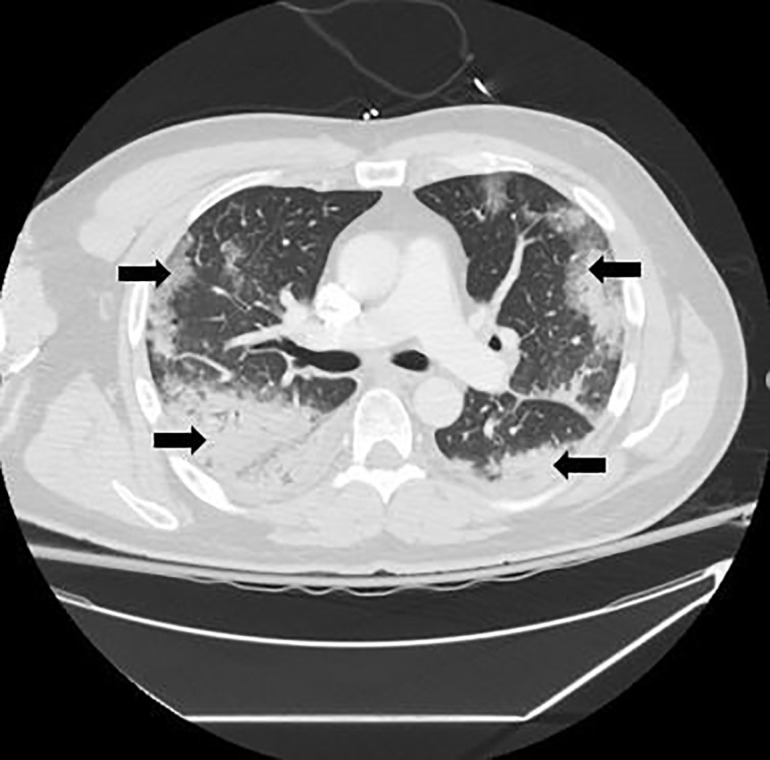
Computed tomography of the chest two days after admission demonstrating extensive, bilateral multifocal pneumonia most notable in the lower lobes (arrows).
